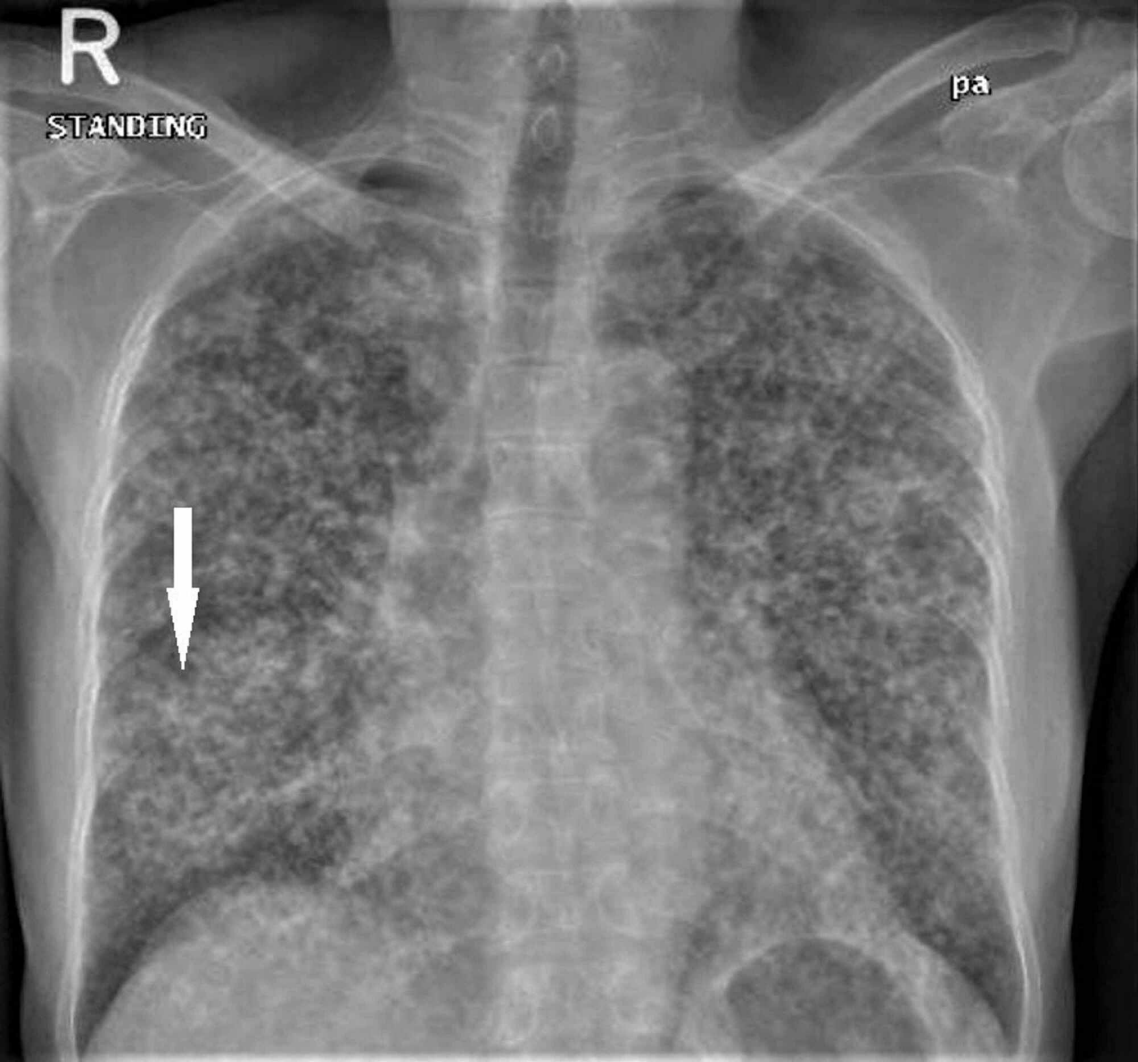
Chest X-Ray (CXR) Posteroanterior (PA) chest X-ray view depicting bilateral miliary shadows and right lung suspicious infiltrates (Arrow).
